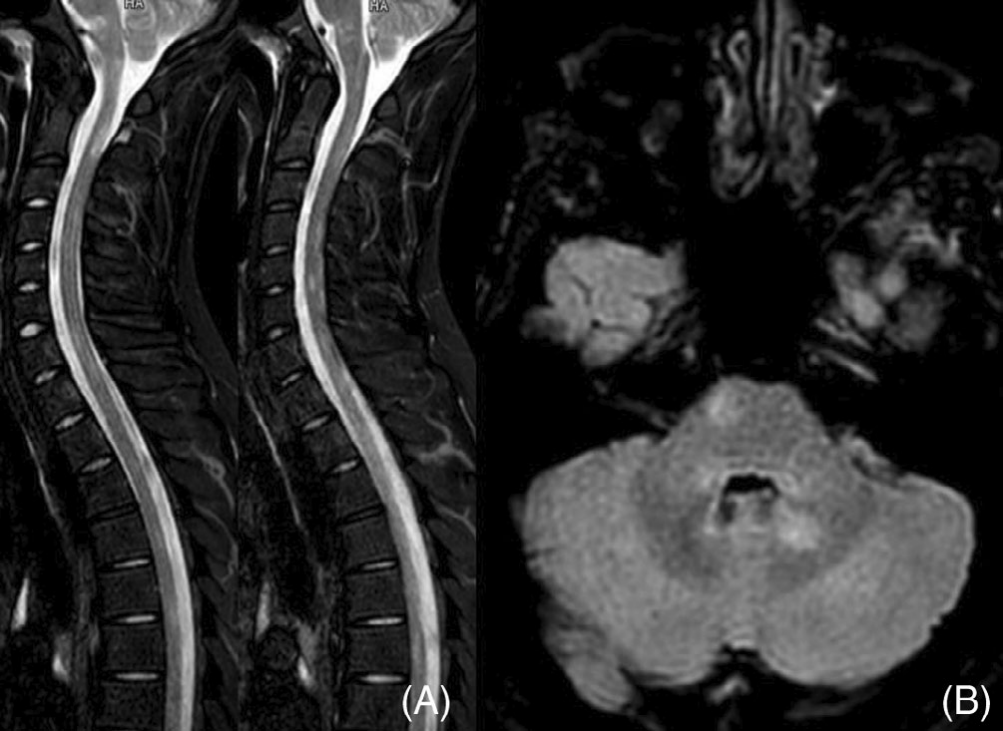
(A) Spinal magnetic resonance imaging (MRI) shows non‐enhancing spinal cord lesions at C2, C4, C5‐C6, T1, T5‐T6, and T10‐T1. (B) Brain MRI, imaging infratentorial region, shows multiple non‐enhancing lesions in the pons and in cerebellar hemispheres
